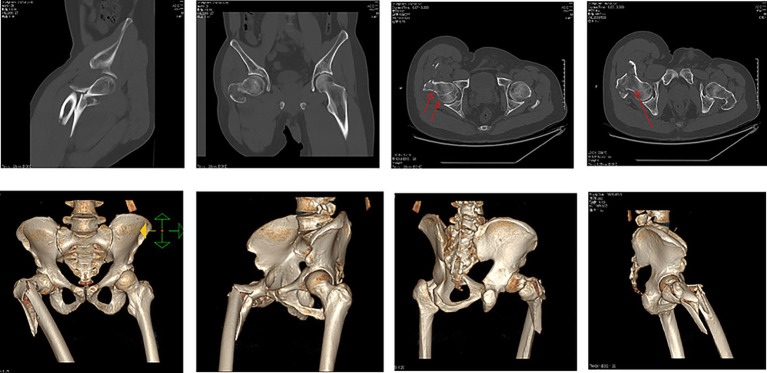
Preoperative pelvic CT scan demonstrating a subtrochanteric fracture with an inter-trochanteric component (Frank–Seinsheimer Type V).
